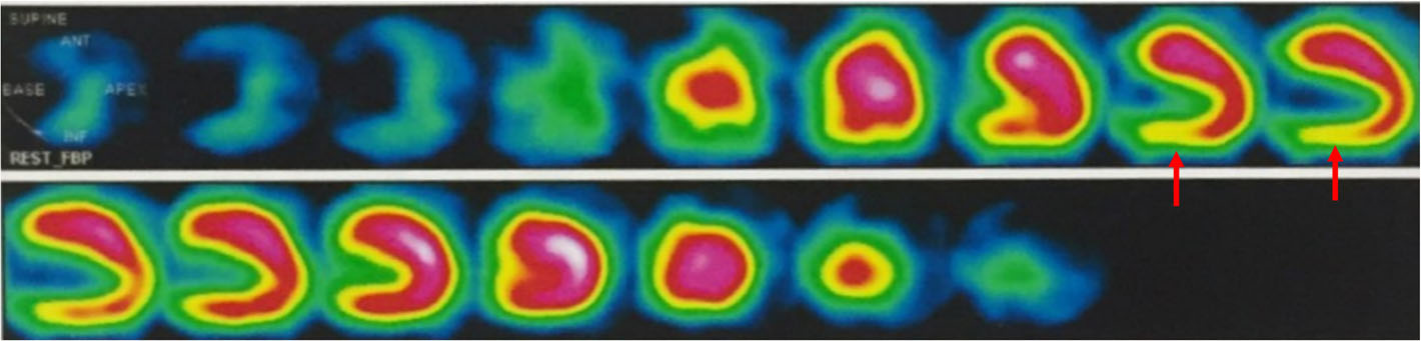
Emission computed tomography revealed mild myocardial ischemia of the left ventricular inferior wall (*red arrowheads*)
